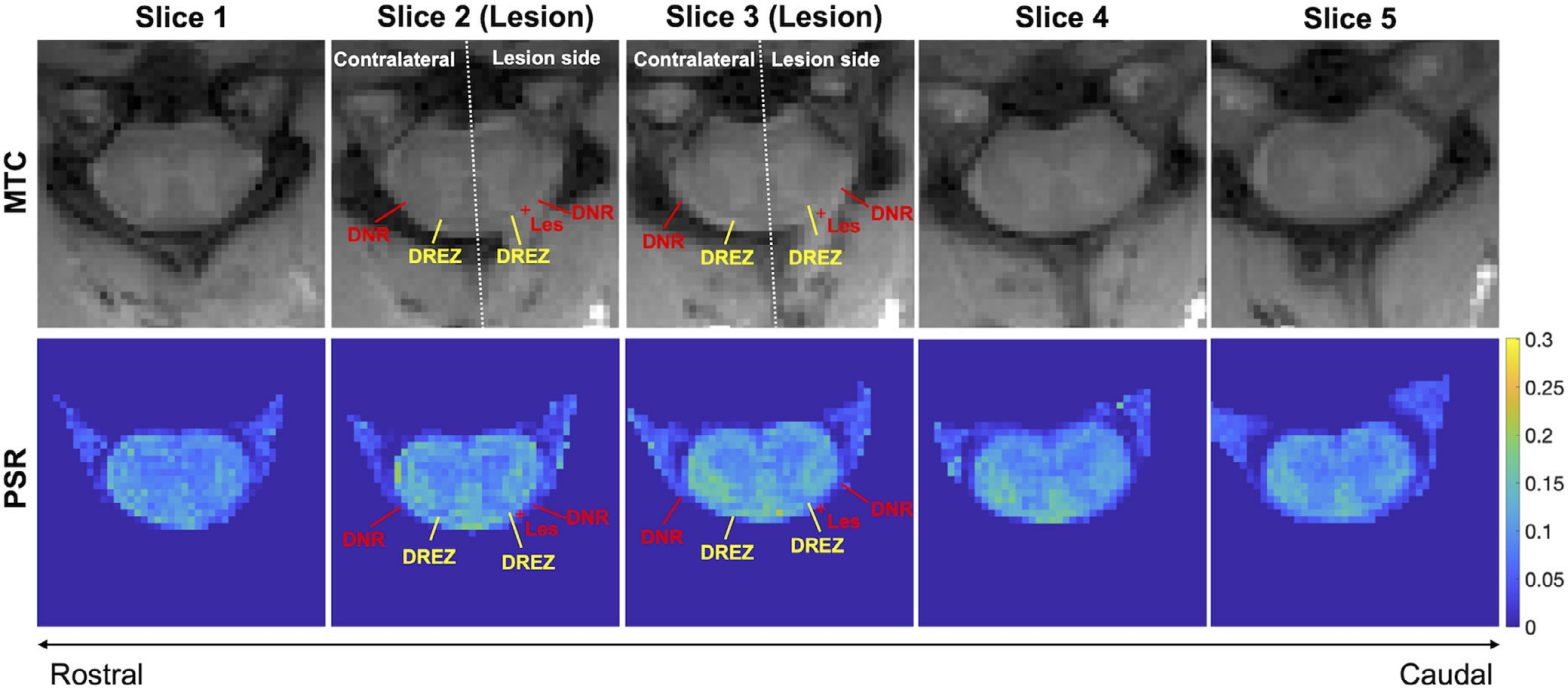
Comparison of pool size ratio (PSR) maps from quantitative magnetization transfer MRI. DNR, dorsal nerve roots; DREZ, dorsal root entry zone; Les, Lesion site; MTC, magnetization transfer contrast, indicated by the red “+” symbol. Unilateral changes in DNR and DREZ regions are evident in PSR maps. Results are from a representative subject following dorsal nerve root section.
